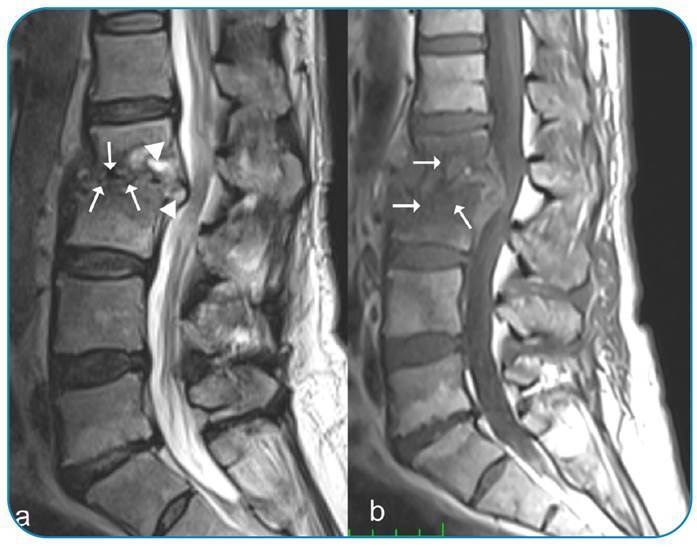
Pretreatment MRI scan. (a) The sagittal T2-weighted image without fat suppression shows erosions (arrow), effusion, and hyperintense signals in the disc space and epidural area (arrowheads) in the lower L2 vertebral and upper L3 vertebral endplates. (b) The sagittal T1-weighted image without fat suppression shows marked signal losses (arrow) in the vertebral bodies and disc space.
